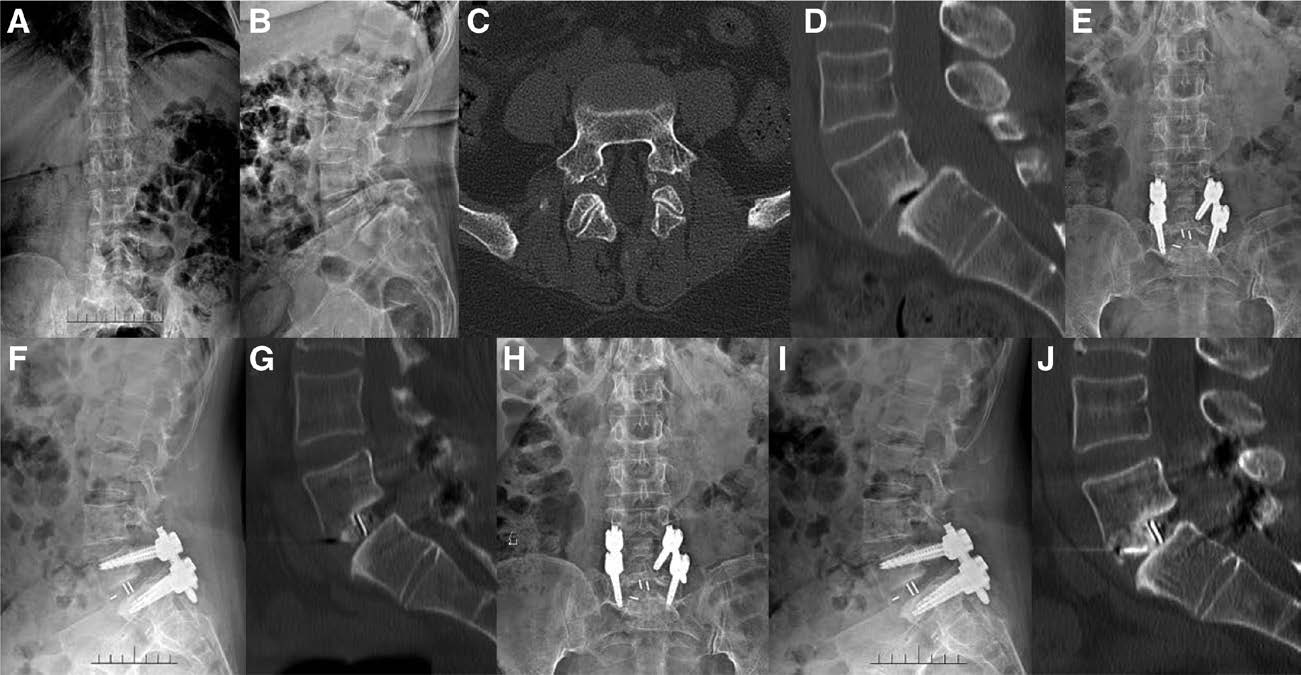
A 63-year-old female patient with bilateral isthmic spondylolisthesis (II°) of the L5 vertebra underwent MIS-TLIF. (A, B) Preoperative X-ray of the lumbar spine shows anterior spondylolisthesis of lumbar 5; (C, D) Preoperative lumbar CT shows bilateral isthmus of lumbar 5 vertebrae, with anterior spondylolisthesis II°; (E, F, G) The X-ray and CT examination 1 day after operation showed that the slippage was reduced satisfactorily and the internal fixation position was good. (H, I, J) X-ray and CT examination 1 year after operation showed good reduction of spondylolisthesis and intervertebral bony fusion. MIS-TLIF = minimally invasive transforaminal lumbar interbody fusion.
